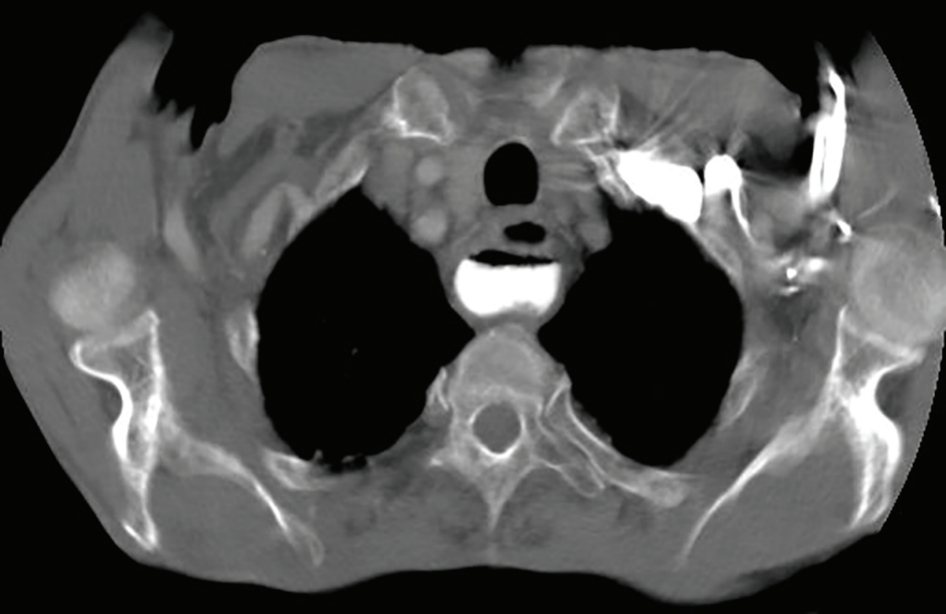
Thoracic CT scan showing the Zenker’s diverticulum.
